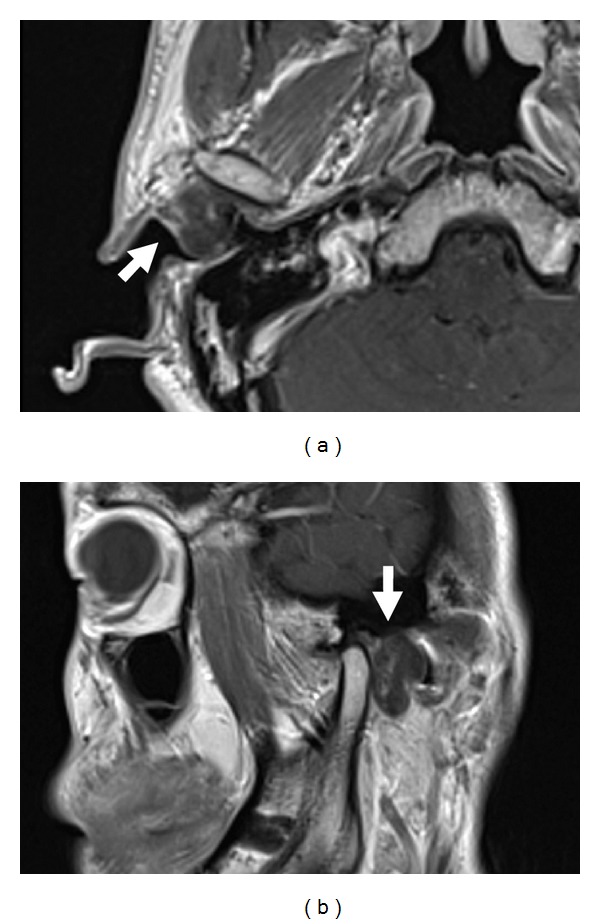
T1 gadolinium-enhanced axial (a) and sagittal (b) magnetic resonance images show a solid tumor (arrow) proximate to the capsule of the right temporomandibular joint in the right preauricular region.
